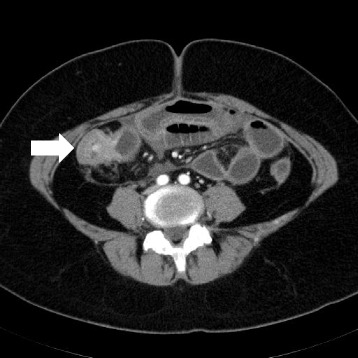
Contrast-enhanced computed tomography (CT). A contrast-enhanced CT image reveals a tumor with contrast enhancement and shows an expanded small intestine
